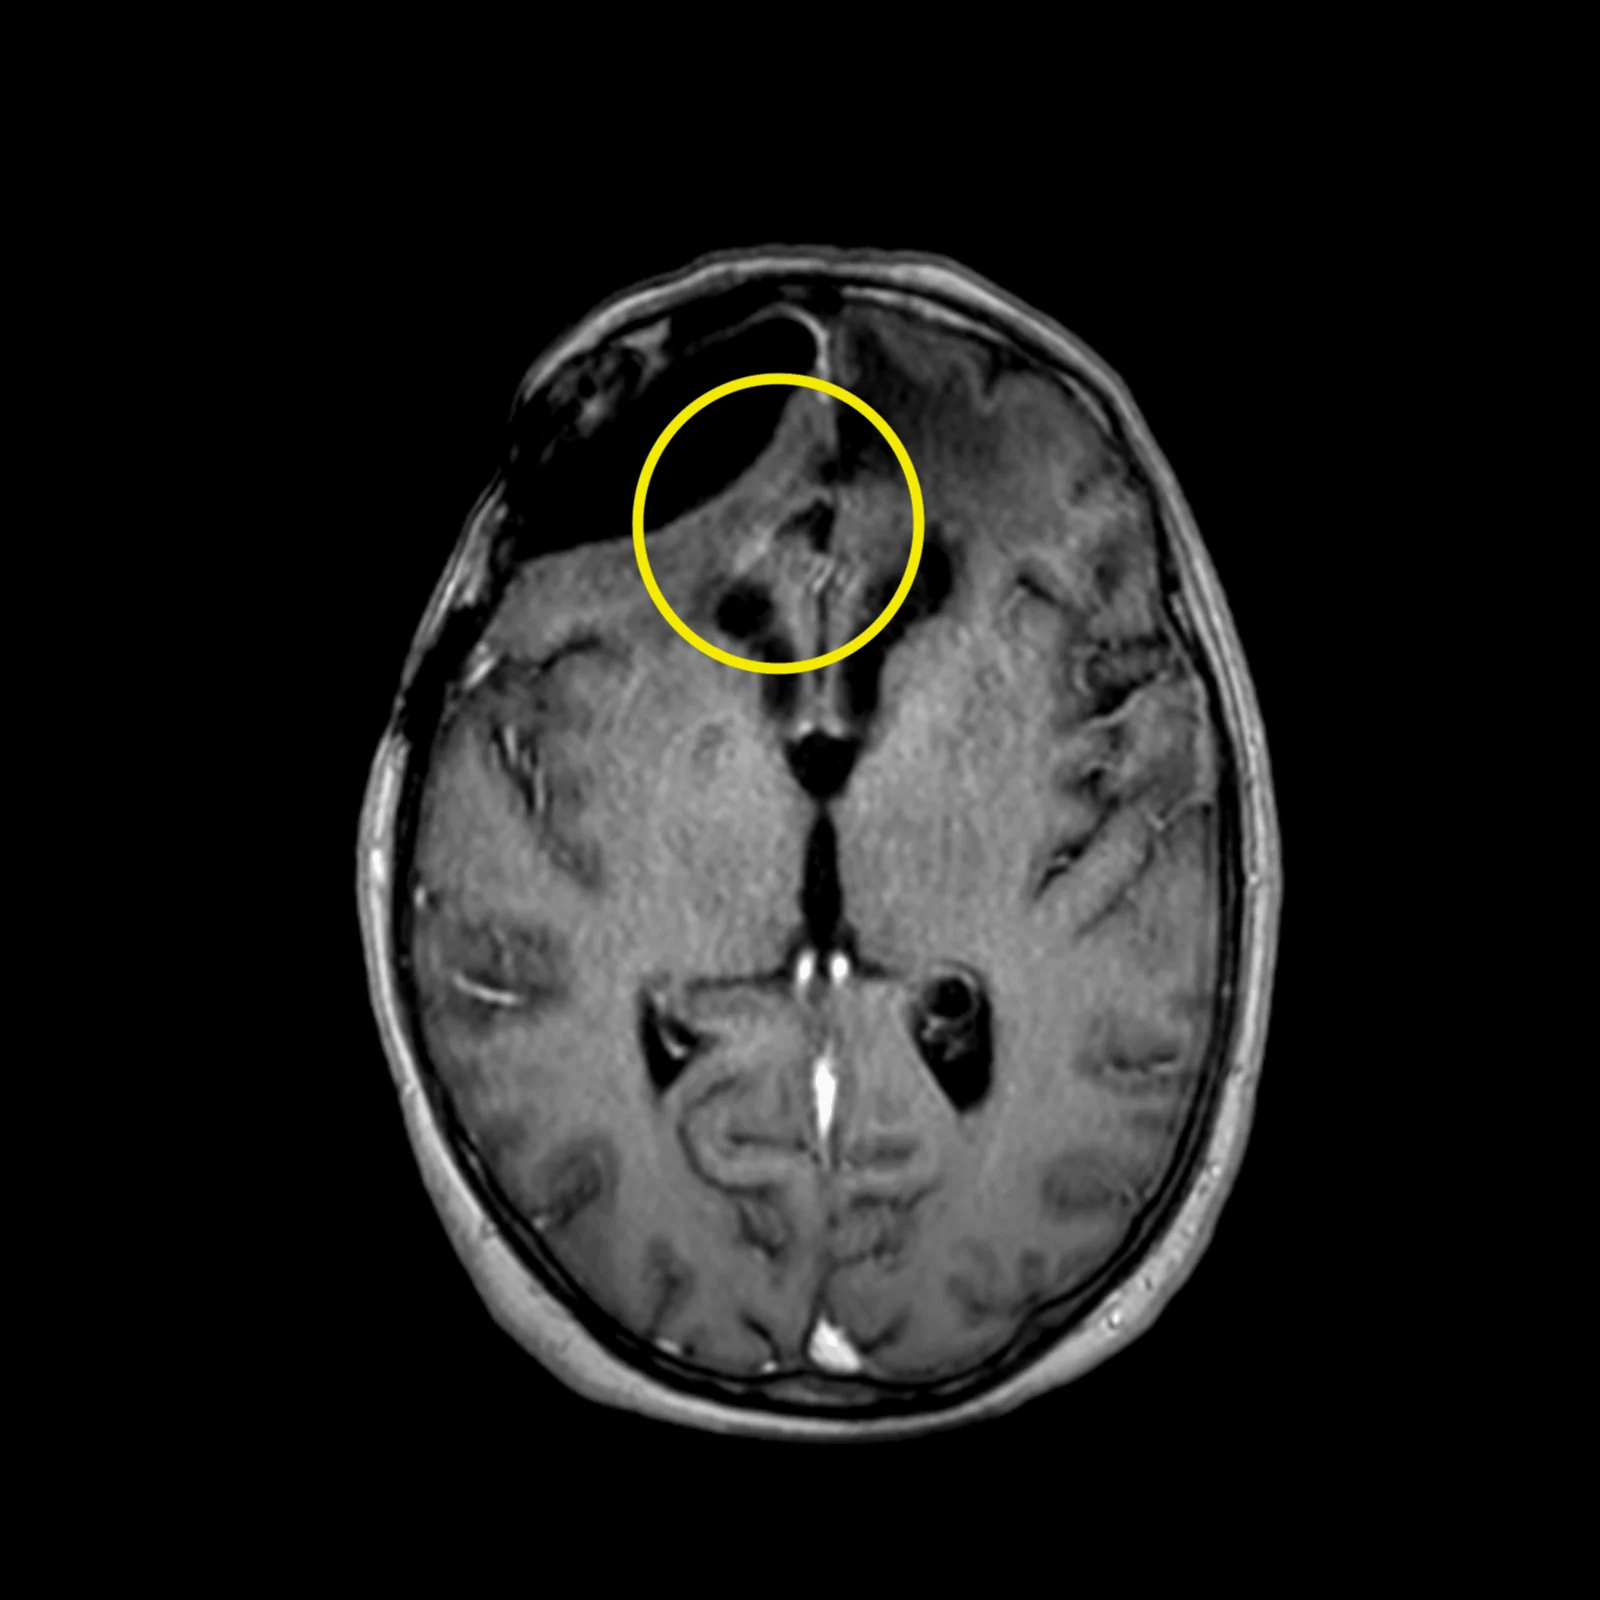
Follow-up post-contrast T1-weighted brain MRI obtained on day 607 Axial post-contrast T1-weighted MRI demonstrating slight interval reduction and stability of the residual left frontobasal enhancing lesion, highlighted by the yellow circle, following adjuvant radiotherapy MRI: magnetic resonance imaging
